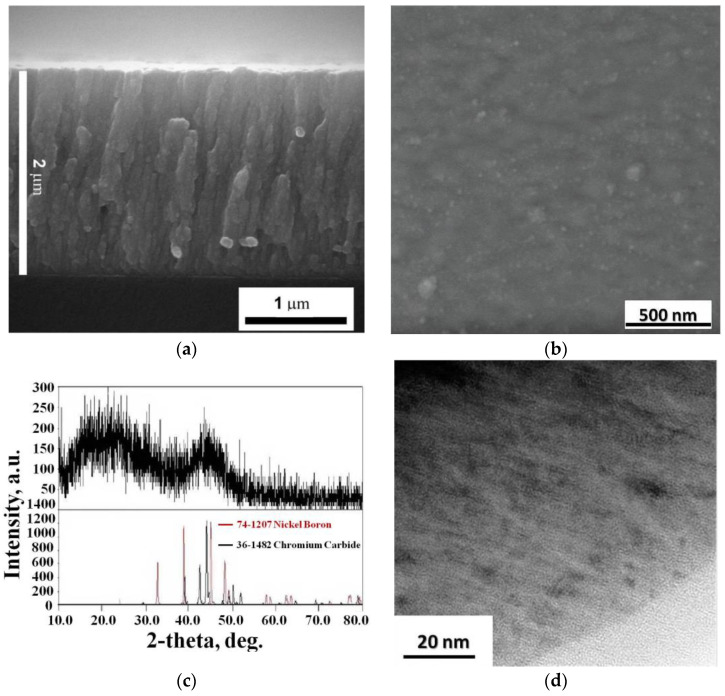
NiB-Cr_7_C_3_ coating on the surface of a 65G steel substrate: SEM image of the fracture (a), and the surface (b), X-ray phase analysis (c), and TEM image (d).
